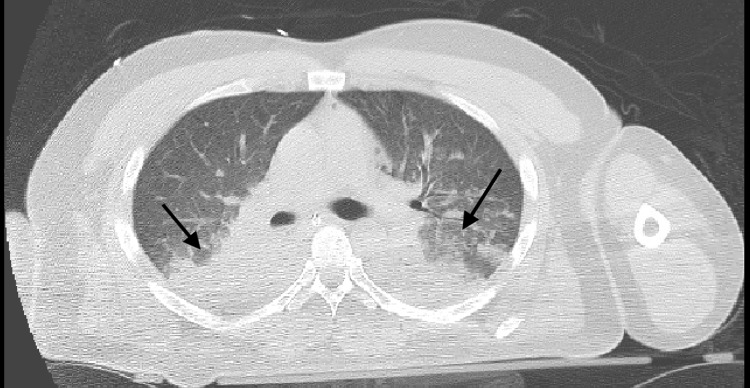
Computed tomography imaging of the chest without contrast demonstrating bilateral pneumonia highly suspicious of COVID-19.
